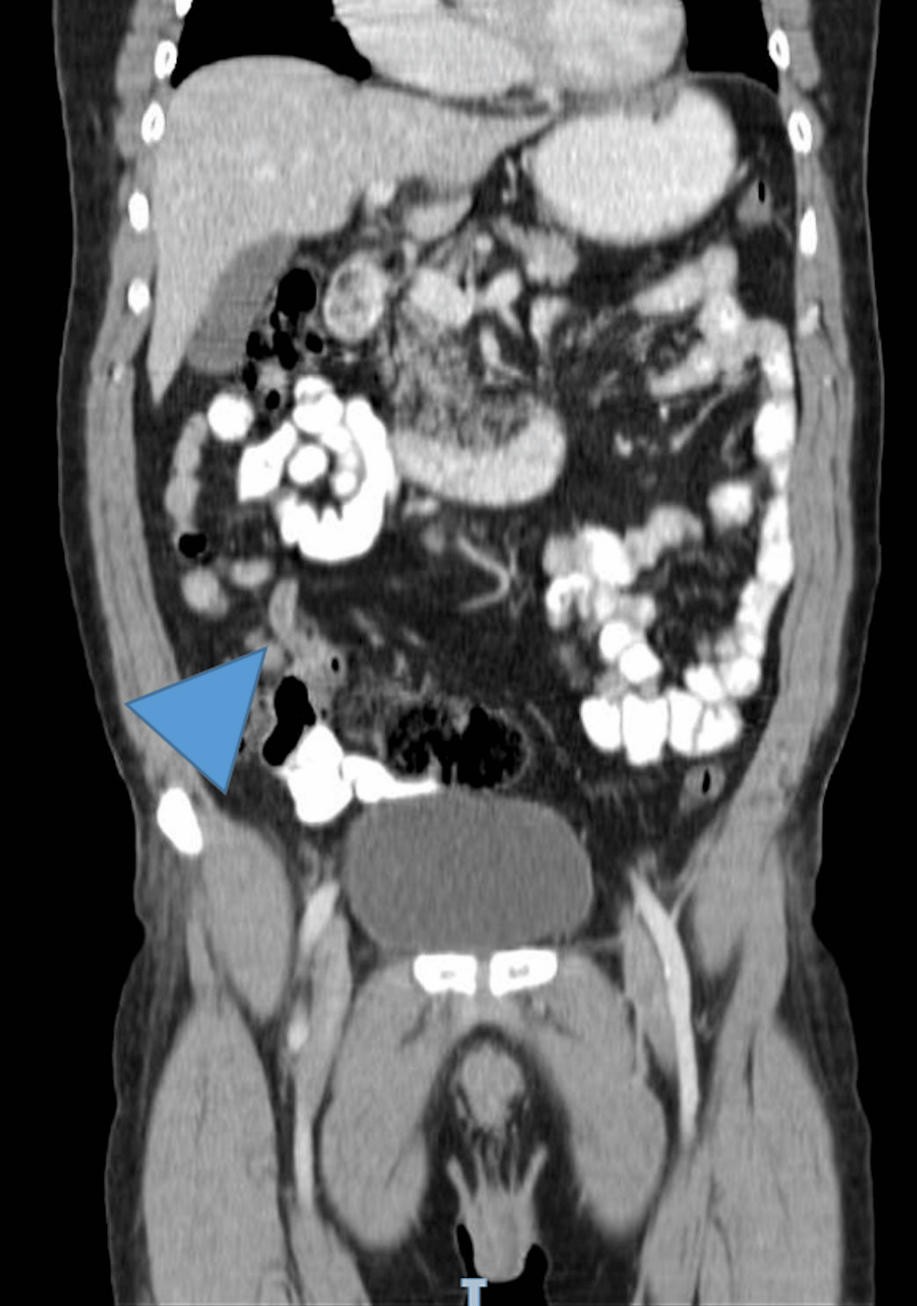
Thickened appendix. CT coronal view showing thickened appendix (arrowhead).
